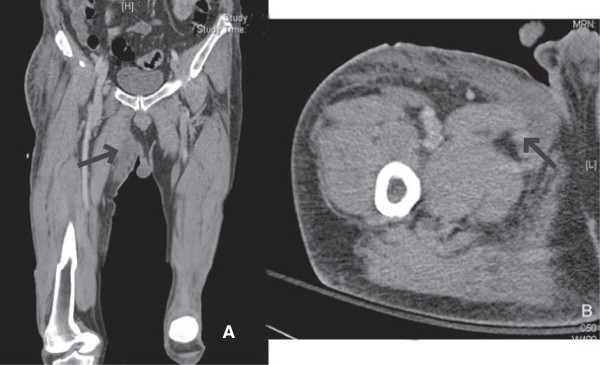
Computed tomography of the pelvic area and right thigh showed formation of an abscess with gas bubbles at the medial portion of the right thigh that involved the underlying adductor muscle. (A), Coronal section, (B), Axial section.
